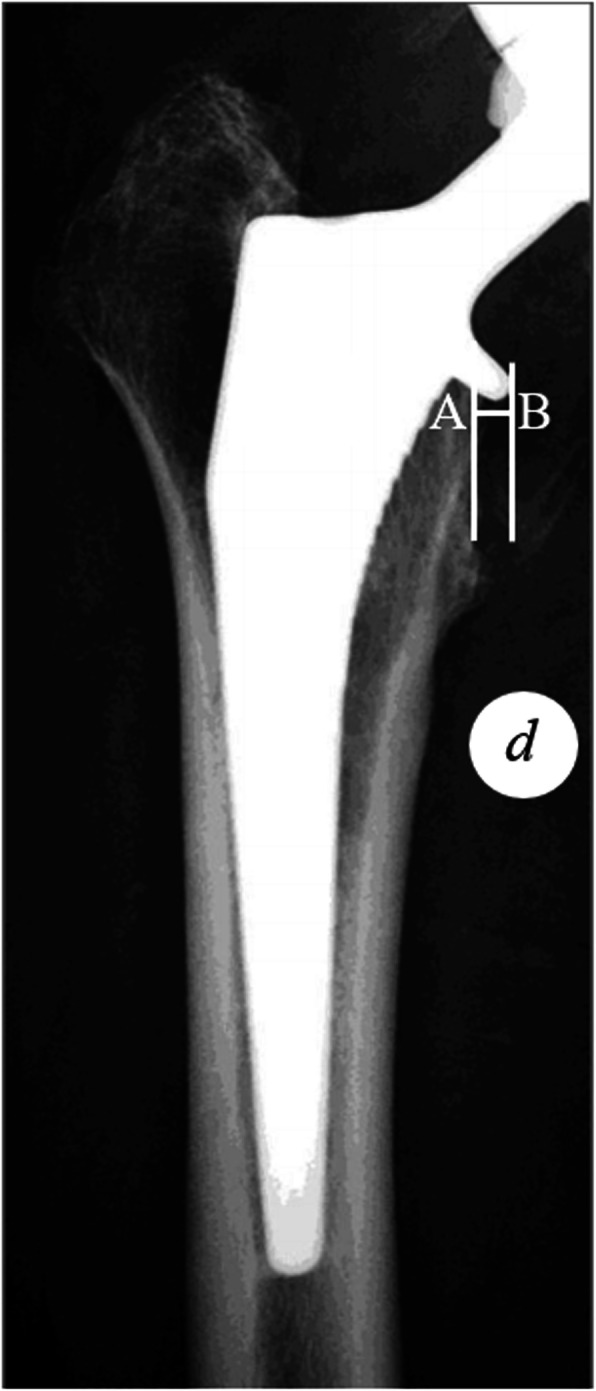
Measurement of collar protrusion length (CPL). Point A represents the medial aspect of the calcar and point B represents the medial aspect of the metal collar. *d* represents the diameter of coin measured on the X-ray. CPL was measured as the distance between the vertical line across points A and B, after correction for magnification. A positive CPL represents overhang of the collar over the calcar
